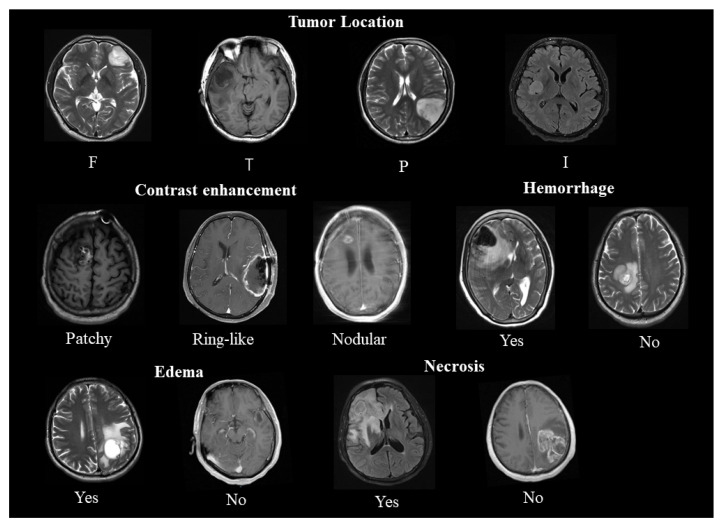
A representative example of conventional magnetic resonance imaging features. *Upper panel:* tumor location (F: frontal, T: temporal, P: parietal, I: insular). *Second panel:* patterns of contrast enhancement (left) and hemorrhage (right). *Lower panel:* edema (left) and necrosis (right).
